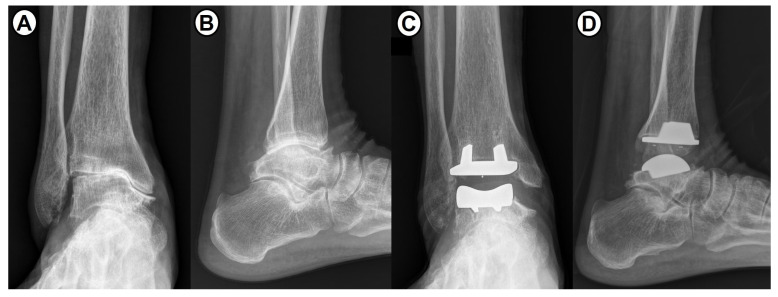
(A,B) Preoperative and (C,D) postoperative radiographs in a 64-year-old male with total ankle arthroplasty for severe osteoarthritis.
